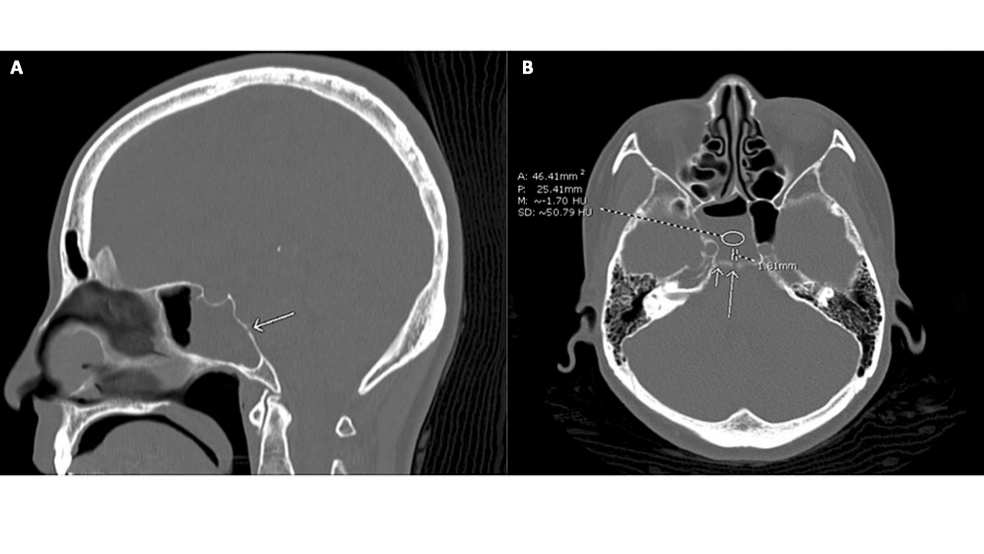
A. Sagittal CT head demonstrating the right clival defect (arrow) and small air-fluid level in right dominant sphenoid sinus. B. Axial CT head demonstrating small air-fluid interface in the right dominant sphenoid sinus, and 1.8 mm (long arrow) and 1 mm (short arrow) defects along the posterior wall of the right sphenoid sinus CT, computerized tomography.
